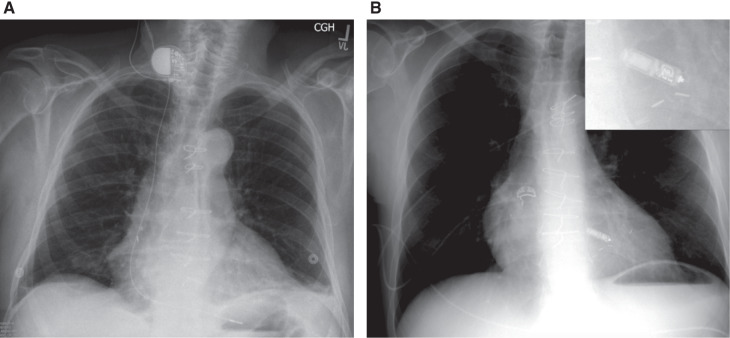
Implanted devices. A: Chest radiograph demonstrating active fixation lead introduced through the right internal jugular vein with an attachment to an externalized PG. B: Chest radiograph demonstrating positioning of the Micra™ device (Medtronic, Minneapolis, MN, USA) (magnified inset).
